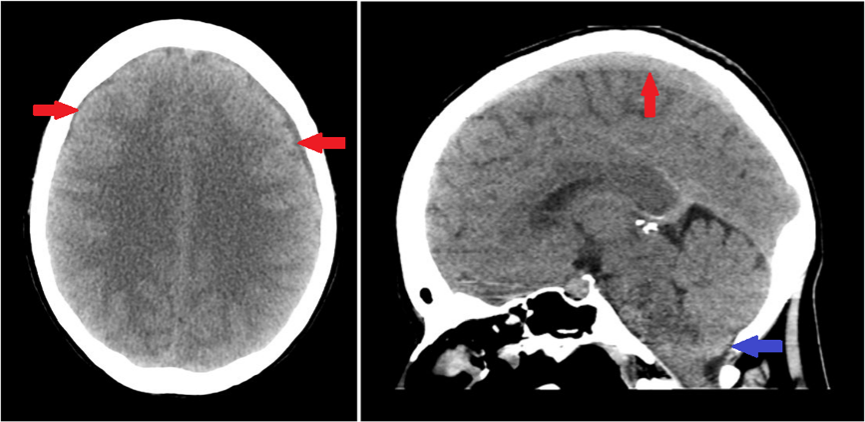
Non-contrast computed tomography head (axial and saggital sections) showing bilateral chronic subdural hematomas (red arrows), with cerebellar descent (blue arrow) but with no evidence of midline shift.
